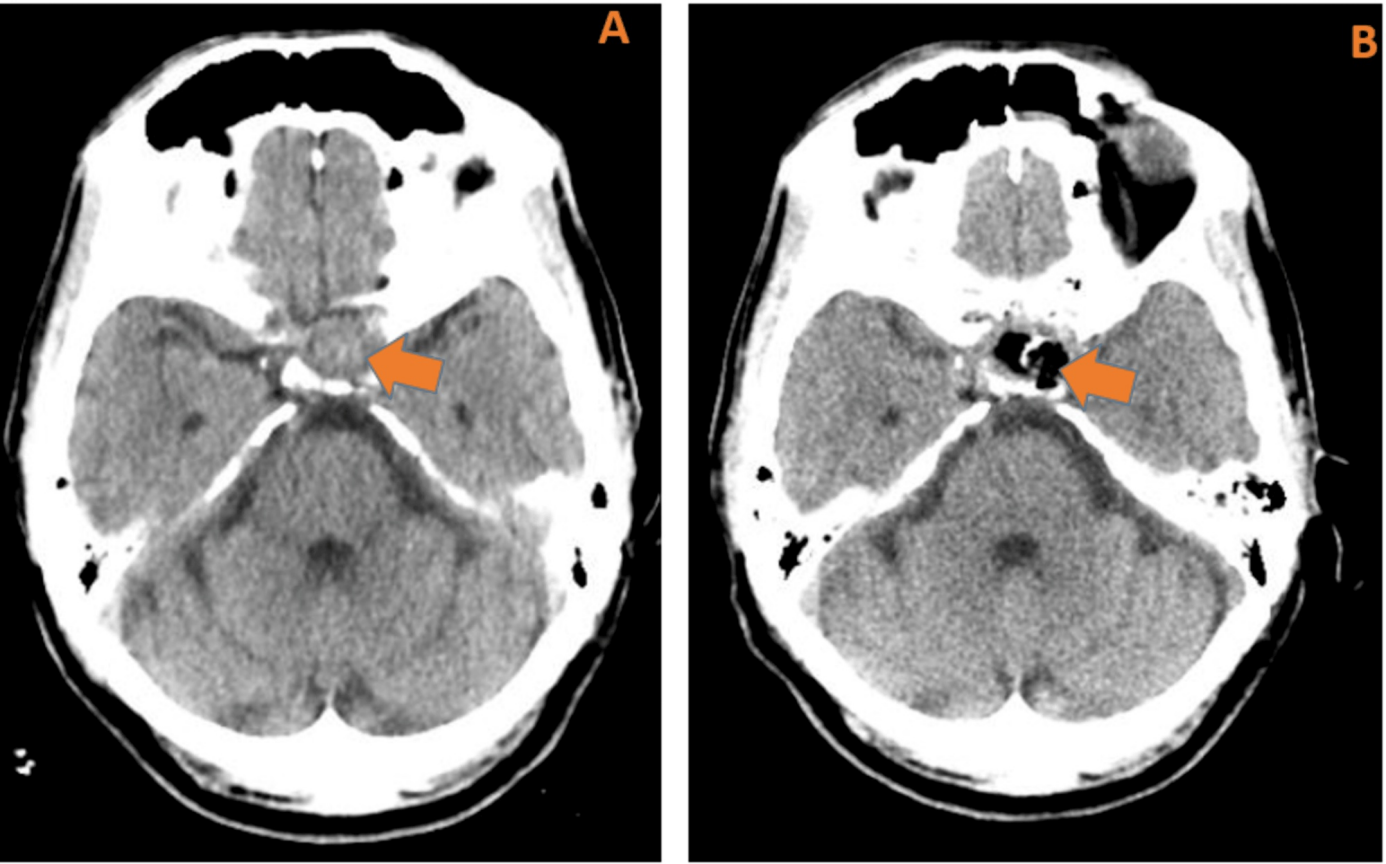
As a comparison with CT head preoperatively (A), CT head without IV contrast, postoperatively (B) showed complete resection of pituitary macroadenoma without a significant residual tumor remaining as shown by orange arrow and associated with small amount of expected left-sided pneumocephalus.
